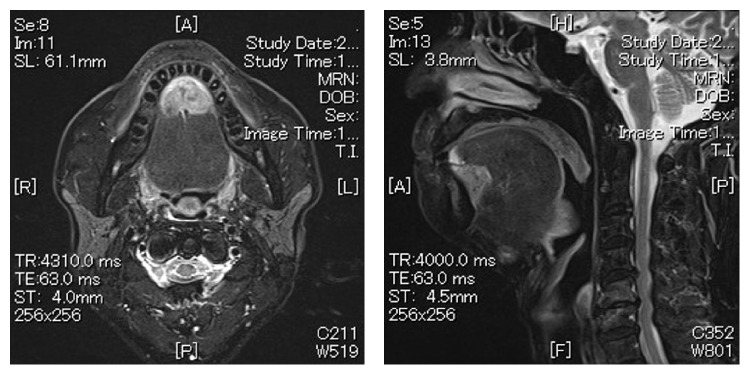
T2 weighted MRI sequence shows a 29 × 23 × 22 mm heterogeneous high signal mass in the floor of mouth.
